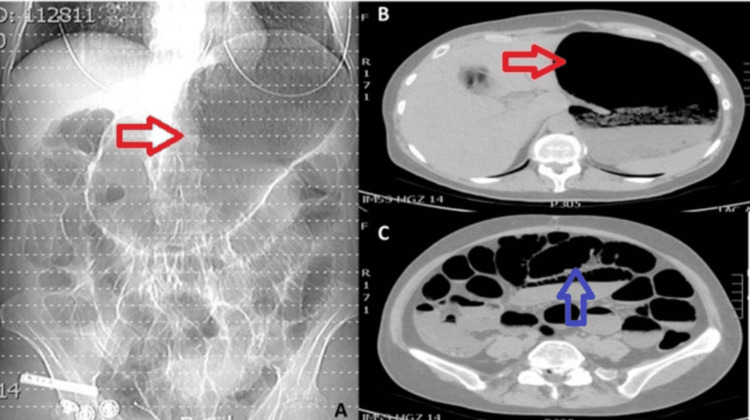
Contrast-enhanced CT of the abdomen and pelvis. (A) Abdominal X-ray showing gastric dilation and dilated bowel loops (red arrows). (B) Simple tomography of the abdomen axial section showing gastric dilation (red arrow) and (C) dilation of intestinal loops (blue arrow).
